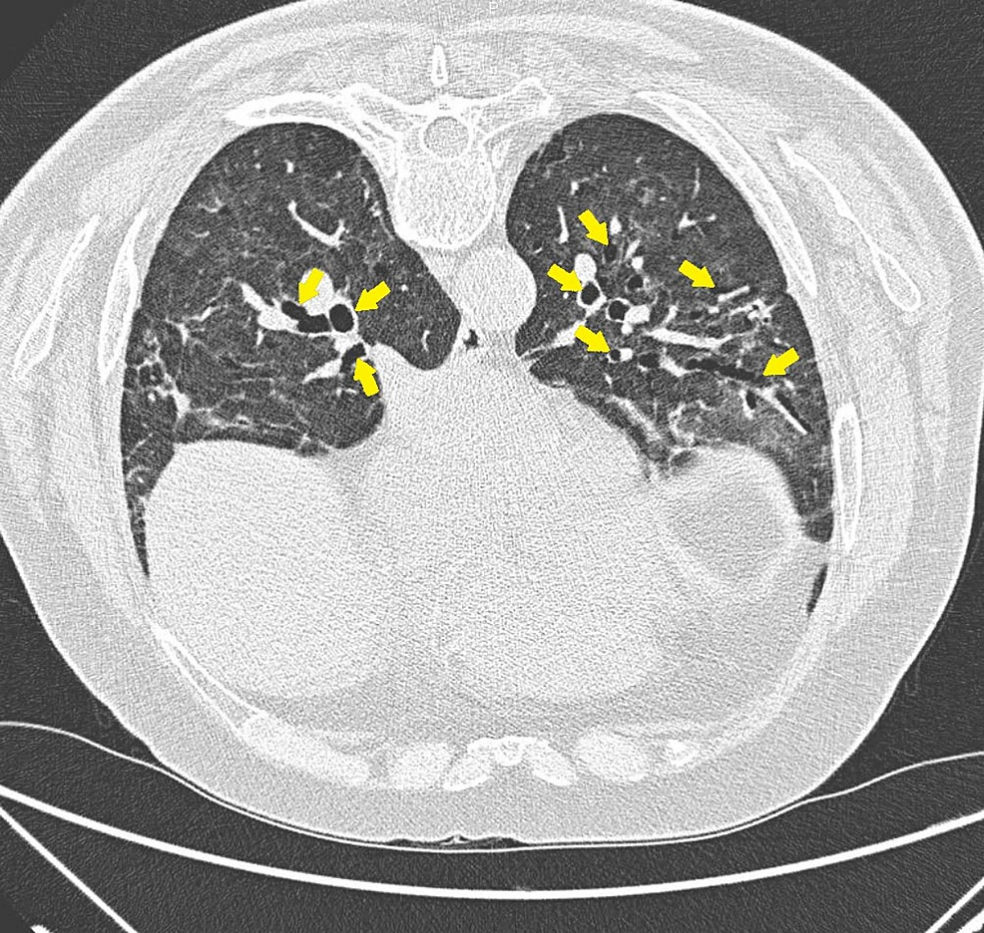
High-resolution CT of the chest (transverse view) at week 16: after five weeks of pirfenidone therapy, there is mild bronchiectasis. There is no reticular nodular density or significant ground-glass opacity. Yellow arrows show bronchiectasis
